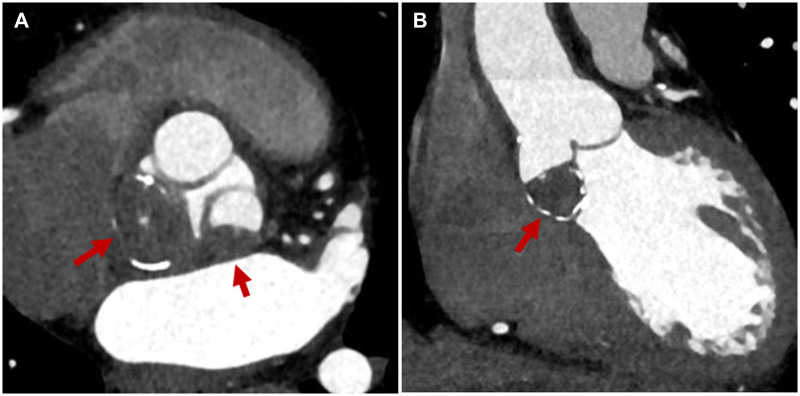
Contrast-enhanced computed tomography. (A) Axial view. (B) Coronal view. A non-enhancing mass surrounded by calcification is seen in the non-coronary cusp and Valsalva sinus and extending into the left-coronary cusp and Valsalva sinus. Red arrow: tumour-like lesion (A color version of this figure appears in the online version of this article).
